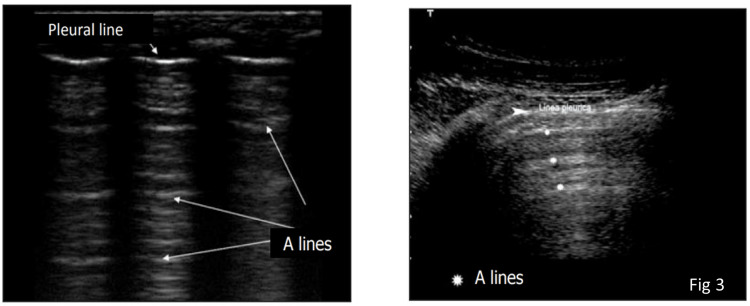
Lung ultrasound images “A” lines on LUS indicate a healthy lung. ”A” lines are horizontal white lines underneath the pleural line. Image source: Reference no. [13]
